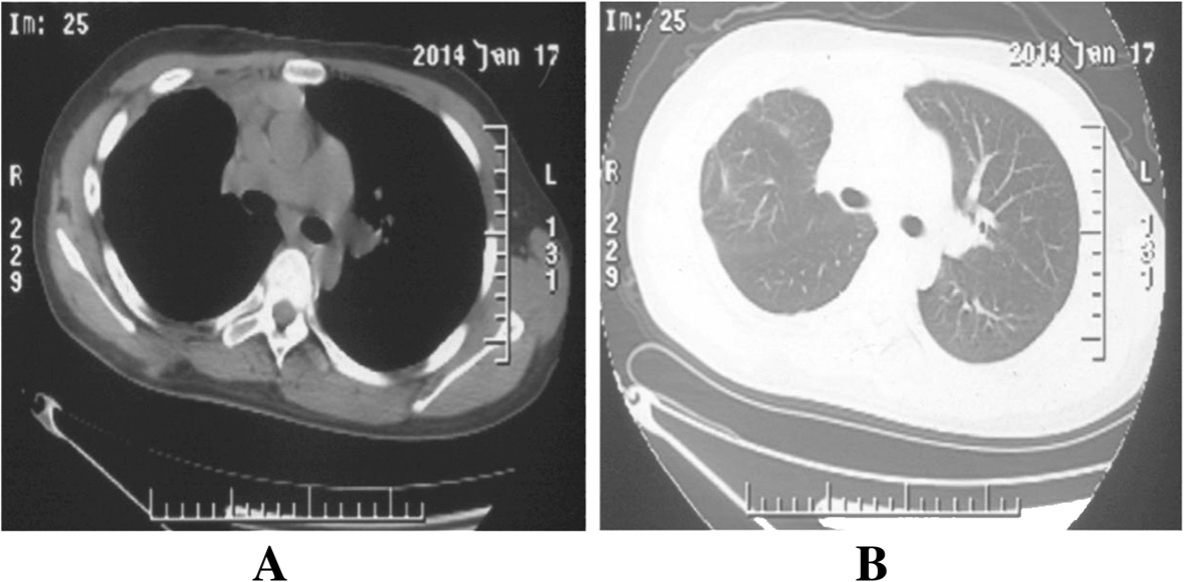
CT scan after operation. (A and B) CT scan 6 months after operation.
